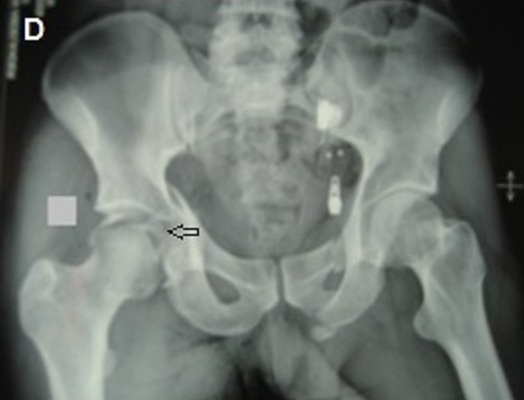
(Patient 2) radiographie du bassin de face montrant une fracture transversale associée à une fracture de la paroi postérieuredu cotyle gauche
